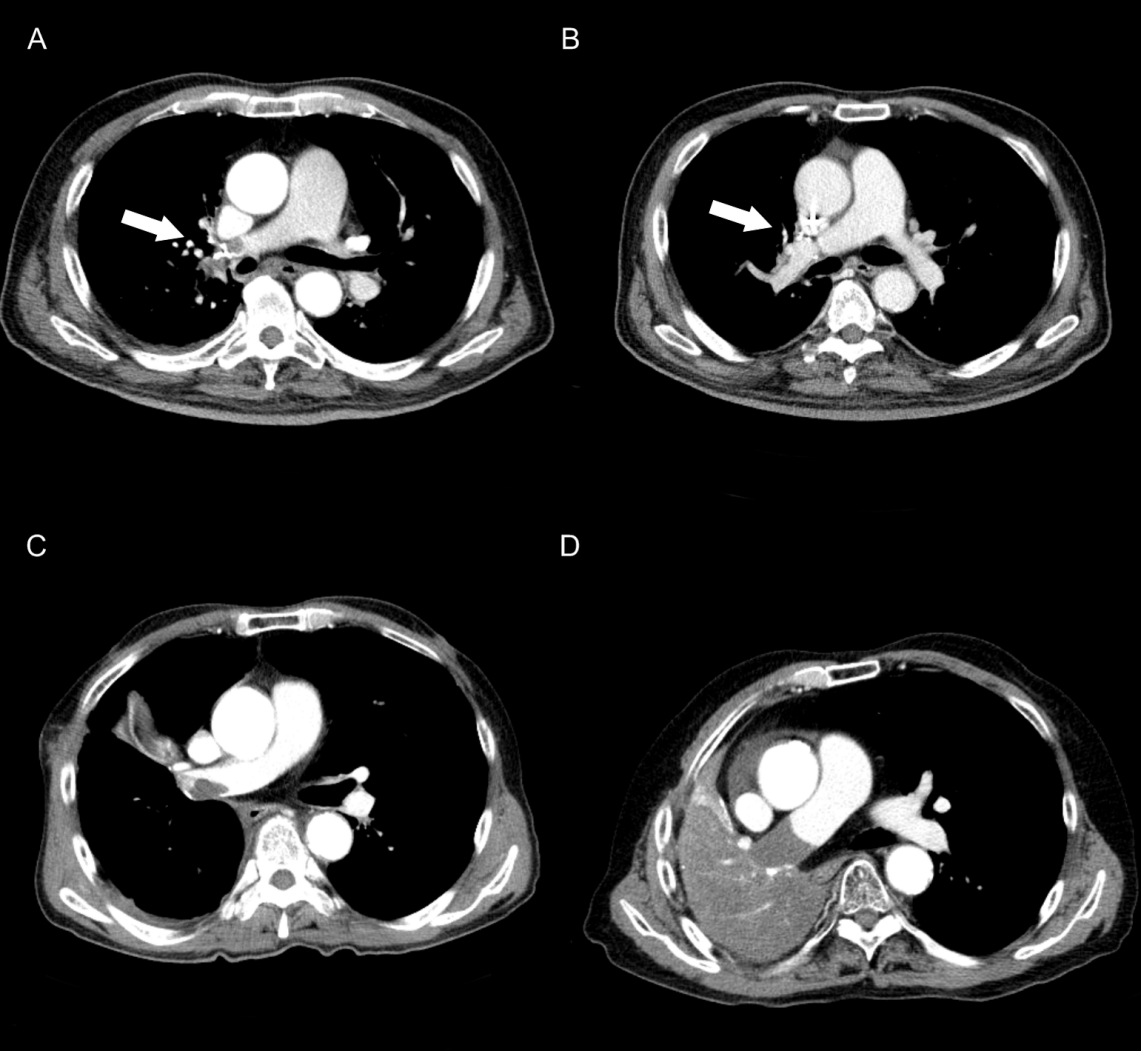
Various courses of vascular stump thrombi on follow-up CT scans. (A) A 77-year-old female status post VATS RUL lobectomy developed pulmonary arterial vascular stump thrombi on the first follow-up CT scan (26 days after surgery). (B) At the second follow-up CT scan, the thrombus had resolved spontaneously (the white arrow indicates the vascular stump). (C) A 72-year-old female status post right middle and lower lobe bilobectomy developed a pulmonary arterial vascular stump thrombus, which was detected 22 days after surgery. (D) The pulmonary arterial stump thrombus first shown as C had grown to completely obstruct the right main pulmonary artery.
